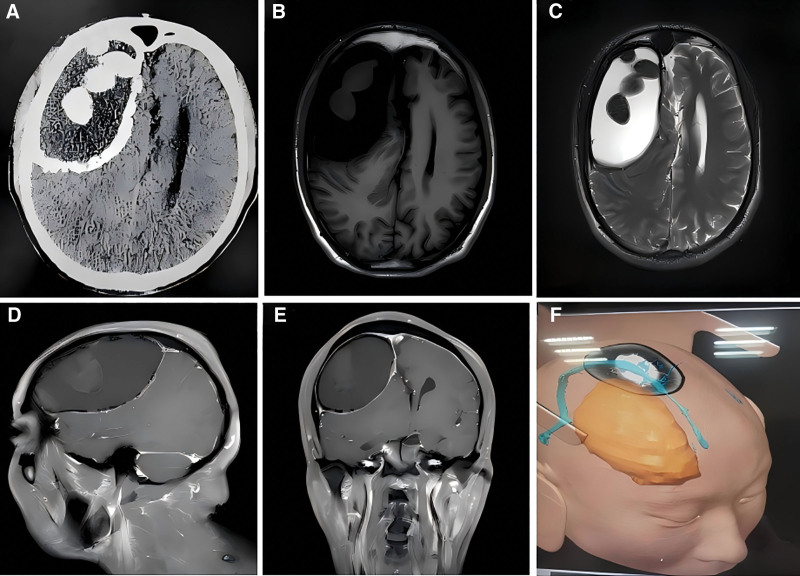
Preoperative cranial CT and MRI examination. (A) Cranial CT scan. (B) Cranial MRI T1-weighted axial image. (C) Cranial MRI T2-weighted axial image. (D) Cranial MRI sagittal image. (E) Cranial MRI coronal image. (F) 3D reconstruction image under neuronavigation. CT = computed tomography, MRI = magnetic resonance imaging.
